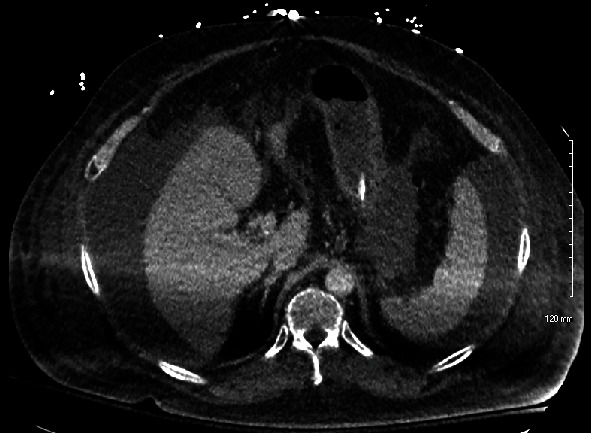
CT angiography chest abdomen pelvis showing radiographic splenomegaly, approximately 13 cm in the anteroposterior dimension.
